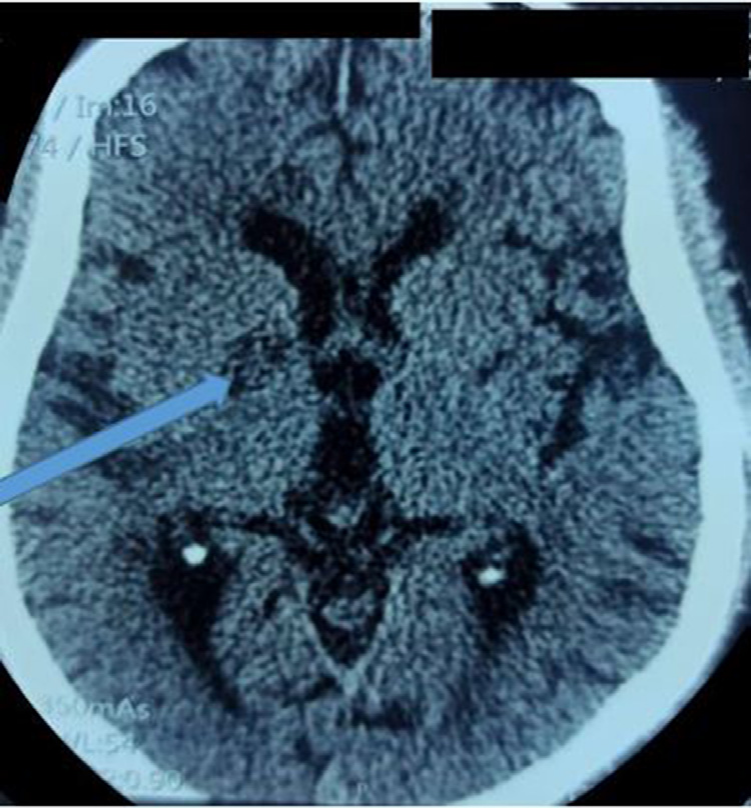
Axial section of brain CT scan without injection showing a right internal capsular infarct.
